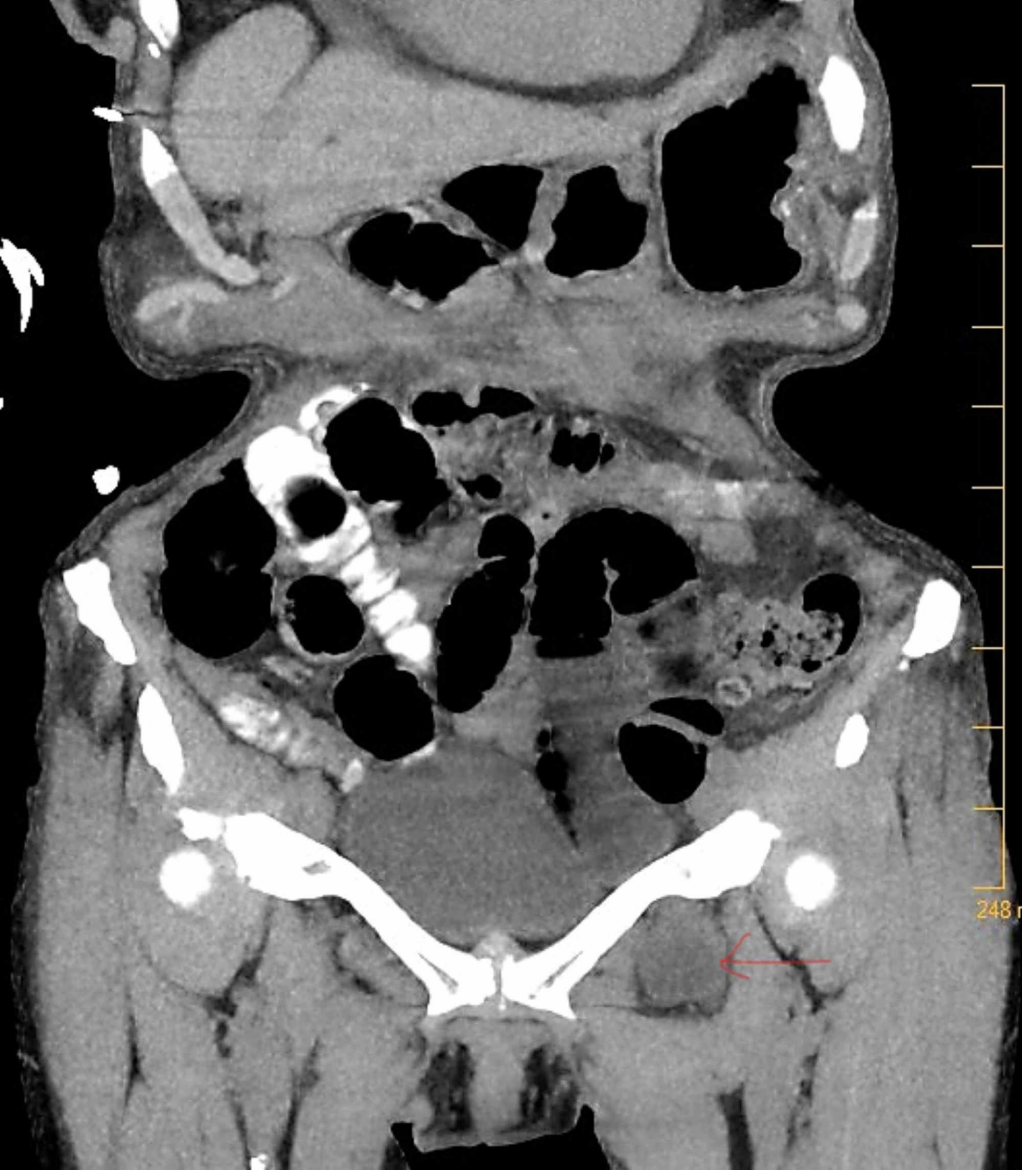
CT scan of the abdomen and pelvis showing the Left Obturator Hernia (Coronal view).
